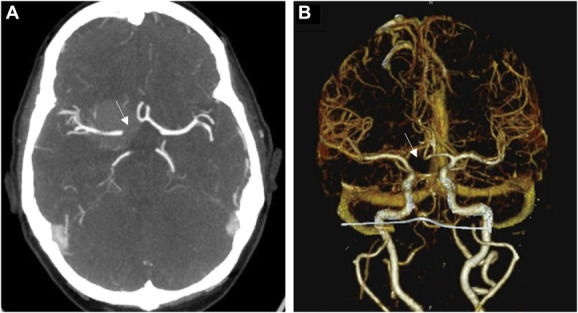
A, Axial view of CTA and B, 3-dimensional reconstruction of CTA demonstrating missing right A1 artery (arrow). CTA, computed tomography angiography.
